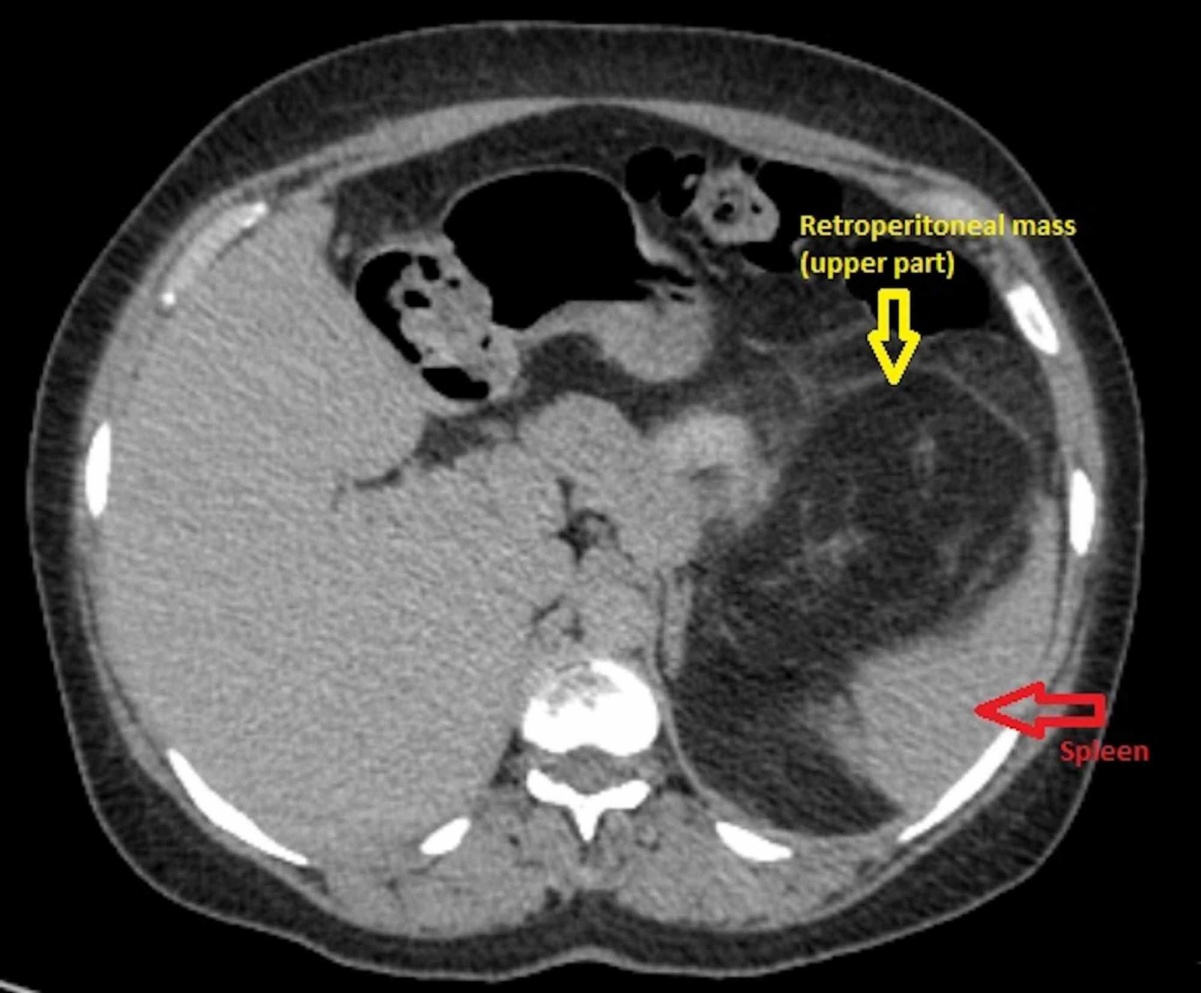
Contrast-enhanced CT scan of the abdomen and pelvis showing a retroperitoneal growth (yellow arrow) displacing the spleen (red arrow) laterally and the stomach medially.
